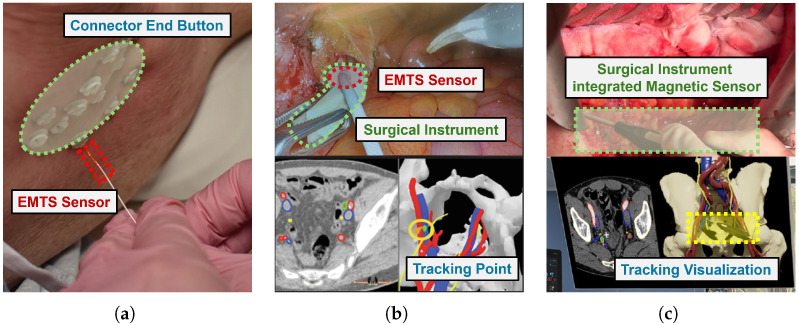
The representative clinical applications. (a) Micro coil-based guidewire used for reconstructing implant geometry. (b) Image-guided navigation with EMTS during robot-assisted surgery. (c) Enhanced CT scan of the patient’s 3D digital model displaying the location of tracked surgical instruments.
